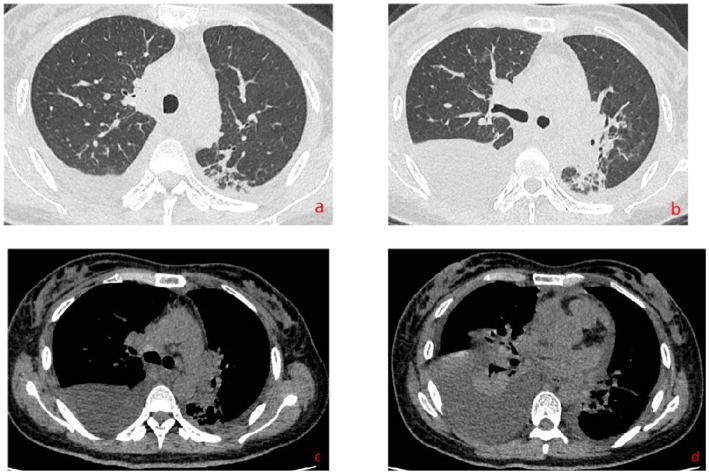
Pulmonary artery CT (November 15, 2024). (a–d) Show no signs of pulmonary artery embolism, uneven thickening of the pericardium and mediastinal soft tissues, and pulmonary vein stenosis. Findings are suggestive of inflammatory changes secondary to fibrinous mediastinitis; metastatic tumors cannot be excluded. Pulmonary lesions and pleural effusion are increased compared to previous findings, with a small amount of pericardial effusion.
